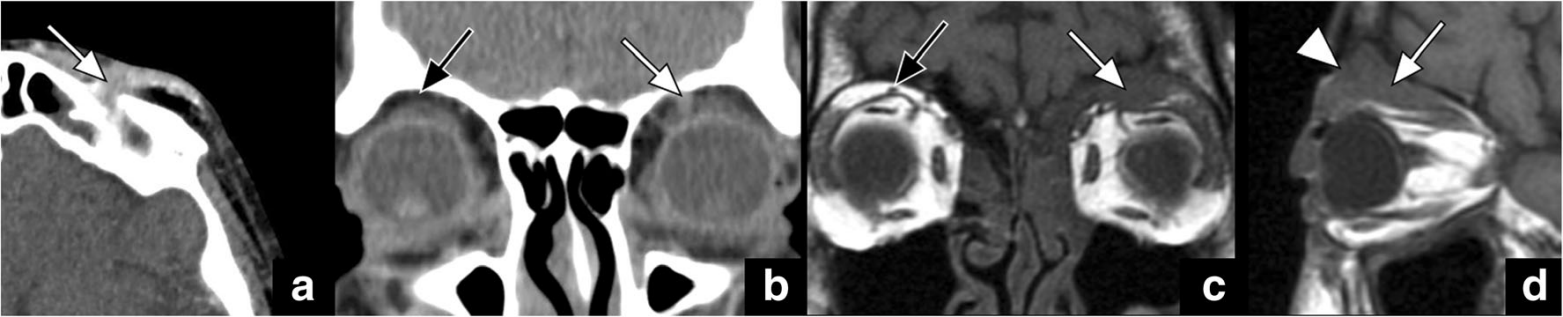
Axial CT (a) and coronal reformatted CT (b) images show obliteration of the fat pad anterior to the superior orbital rim (*arrow* in a) and superior to the levator palpabrae muscle (*white arrow* in b) when compared to its normal appearance (*black arrow* in b). The coronal (c) and sagittal (d) T1 weighted images in a different patient reveal obliteration of the fat pad superior to the left levator palpebrae muscle (*white arrows* in c and d) in the expected location of V1, compared to the normal fat pad on the right side (*black arrow* in c). Notice also the obliteration of the fat pad anterior to the supraorbital foramen (*arrowhead* in d). These signs are indicative of PNTS along V1 in both of these patients with a history of excised skin cancer on the forehead and V1 numbness
